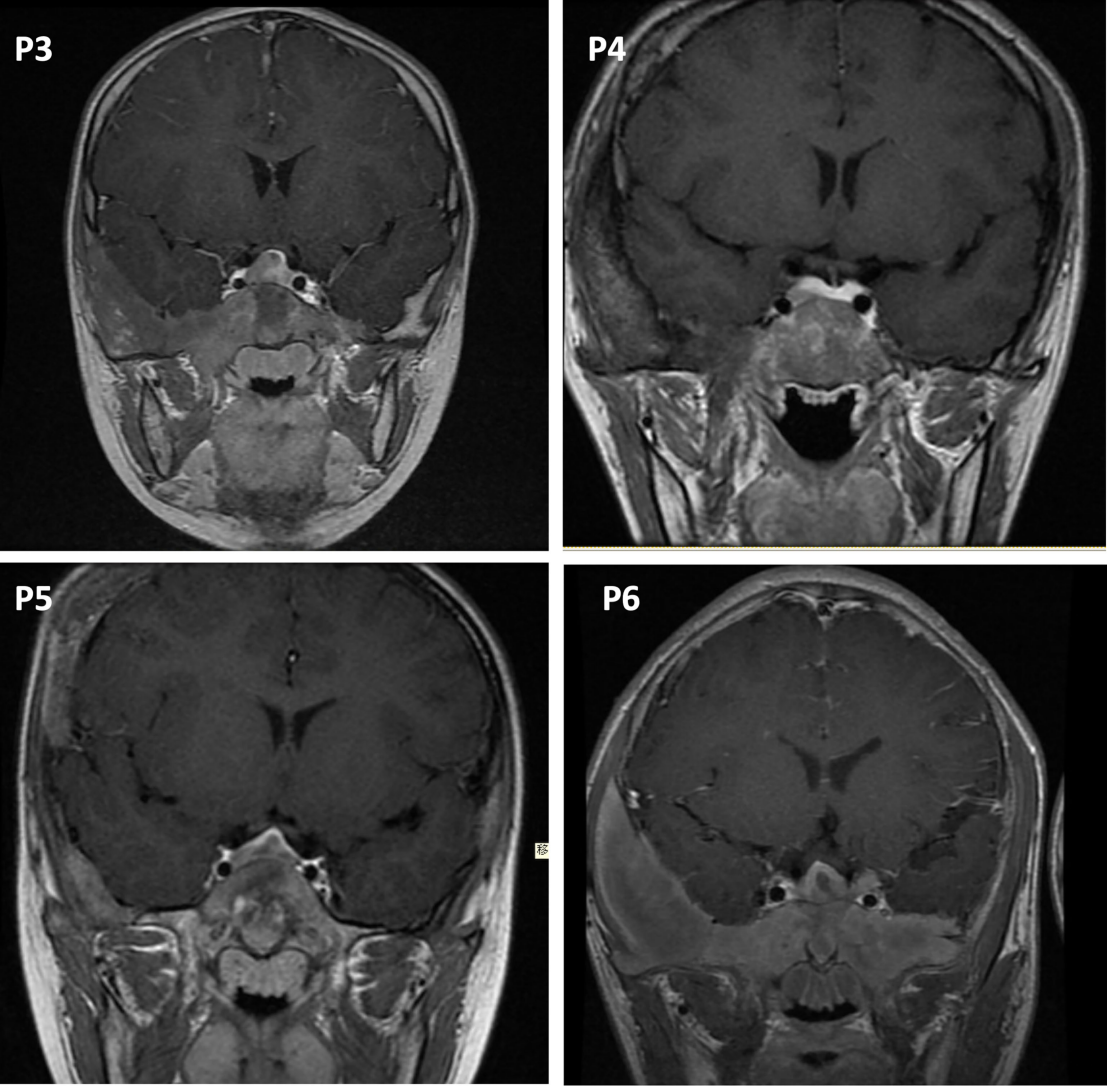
Pituitary MRI in the coronal view. P3, patient 3, macroadenoma. P4, patient 4, microadenoma. P5, patient 5, multiple microadenomas. P6, patient 6, macroadenoma with a cystic zone.
